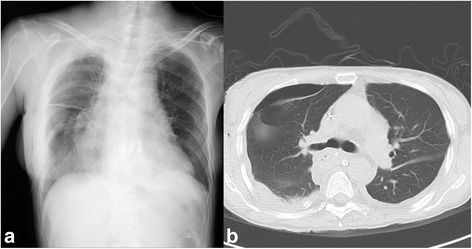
Chest radiography (a) and computed tomography (b) images of the patient on the 3rd postoperative day. a Chest drainage tube, permeability decay, and expansion failure on the right lung fields, subcutaneous emphysema on the right chest wall, drainage tube and gastric conduit on the mediastinal field, and peripheral venous nutrition tube on the left arm are seen. b Chest drainage tube, moderate pleural effusion, and expansion failure on the right lung, and small pleural effusion on the left lung are seen
